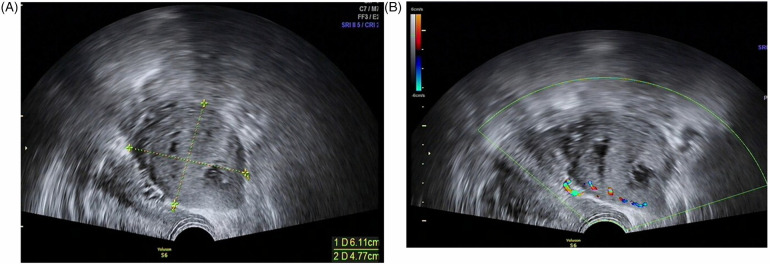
Ultrasound images from a 15-year-old girl with a confirmed ovarian sclerosing stromal tumor. (A) Conventional ultrasound image shows a 6.1 cm smooth outer contour solid lesion with non-shadowing artifact (calibers). (B) Color Doppler image shows minimal vascularity (Color score = 2). The mass was classified as O-RADS 4.
